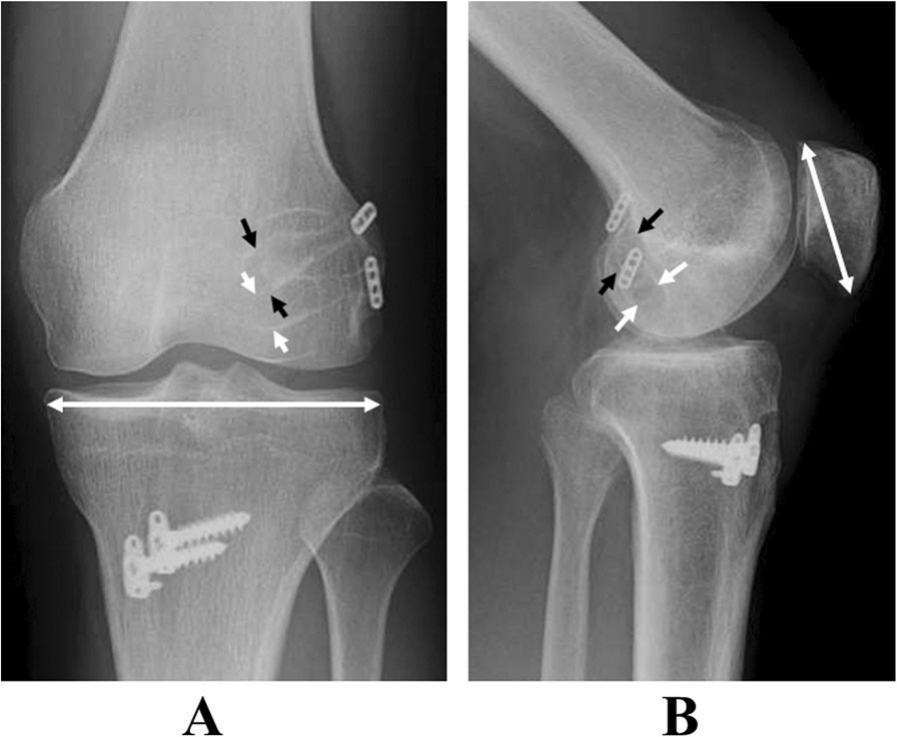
Computed digital radiographs of the knee with double-bundle ACL reconstruction. The two black arrows show the femoral outlet of the anteromedial tunnel, while the two white arrows show the femoral outlet of the posterolateral tunnel. a anteroposterior view 12 months after surgery. b lateral view 12 months after surgery
